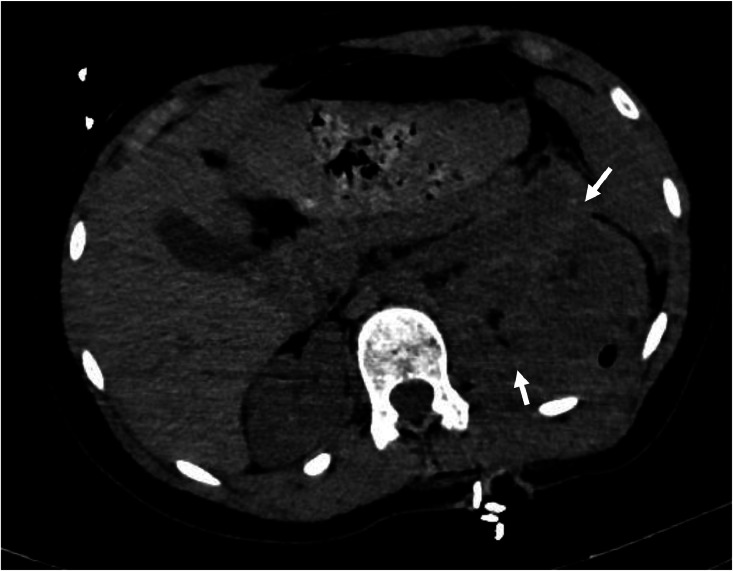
Axial non-contrast abdominal CT showing a large perirenal and pararenal hematoma extending into the intraperitoneal space, consistent with active bleeding.
